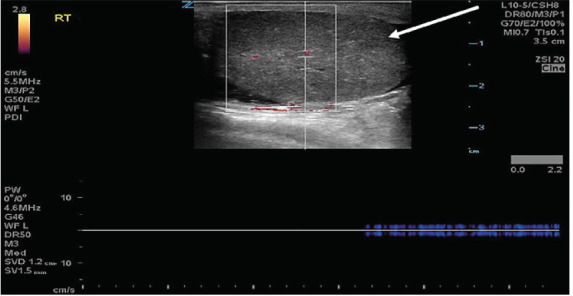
Point-of-care ultrasound demonstrating heterogenous testicle (arrow) with limited, attenuated arterial flow on spectral Doppler.
